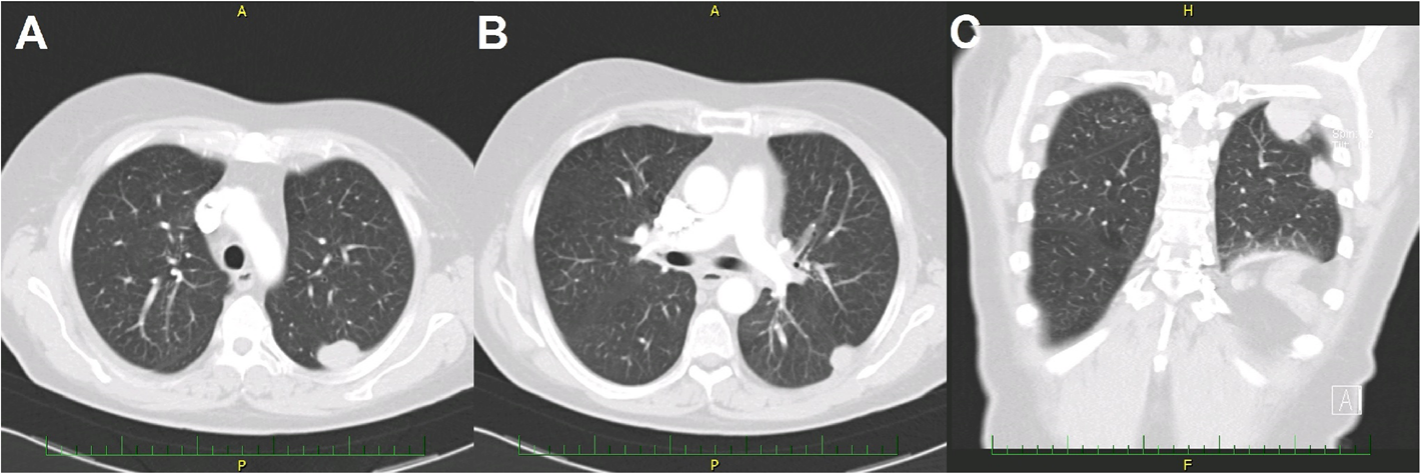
CT scan shows two nodular masses of parietal pleura (a, b – transversal section, c – frontal section)
